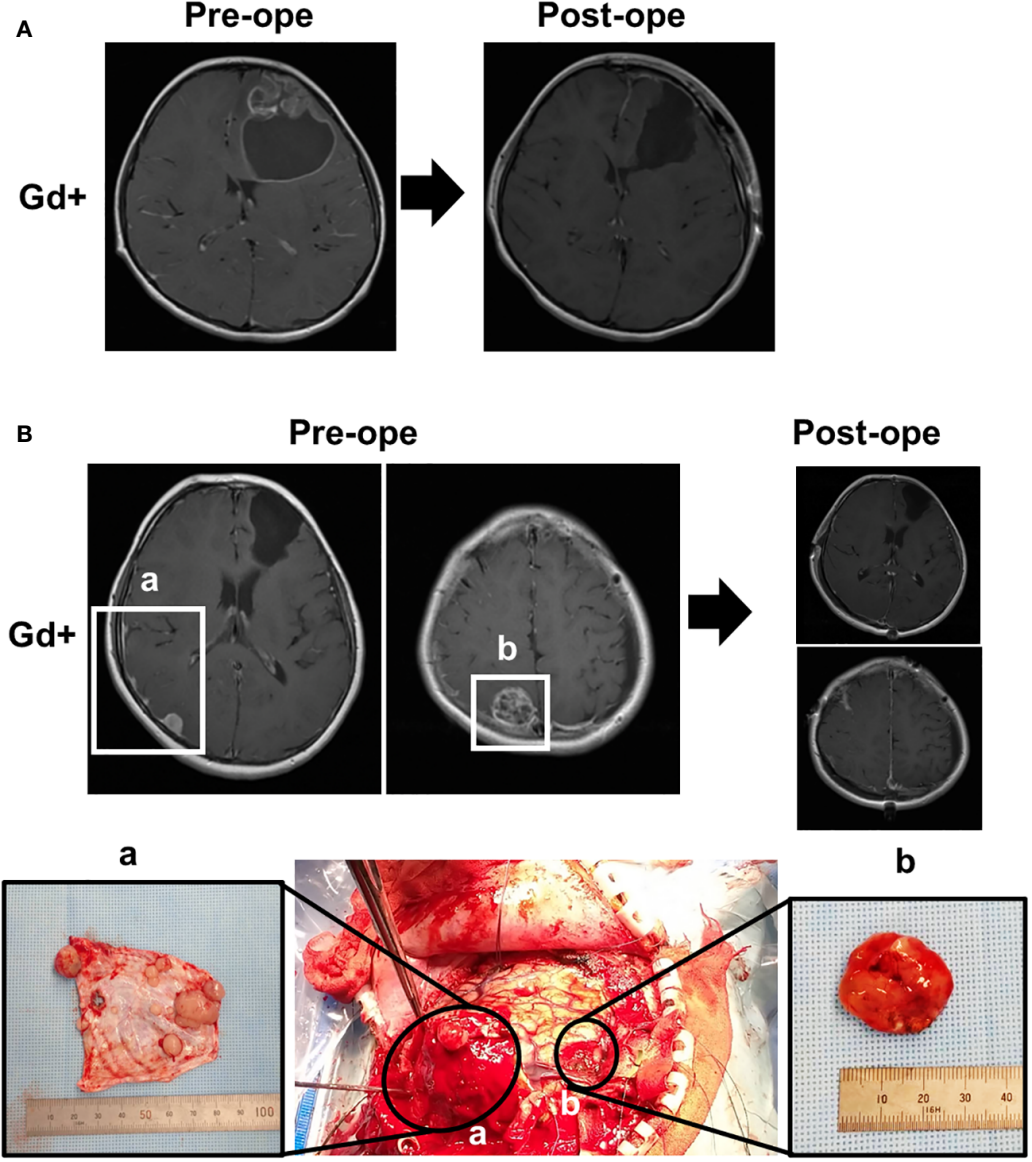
Magnetic resonance imaging (MRI) and intraoperative findings. (A) Preoperative and postoperative MRI at initial presentation, showing a partially contrast-enhancing lesion in the left frontal lobe with a cyst, which was completely removed by surgery. (B) Preoperative and postoperative MRI at the time of recurrence and correspondence with intraoperative findings. (a) Multiple small, enhanced lesions adhering to the dura, and (b) the largest heterogeneously enhanced lesion.
